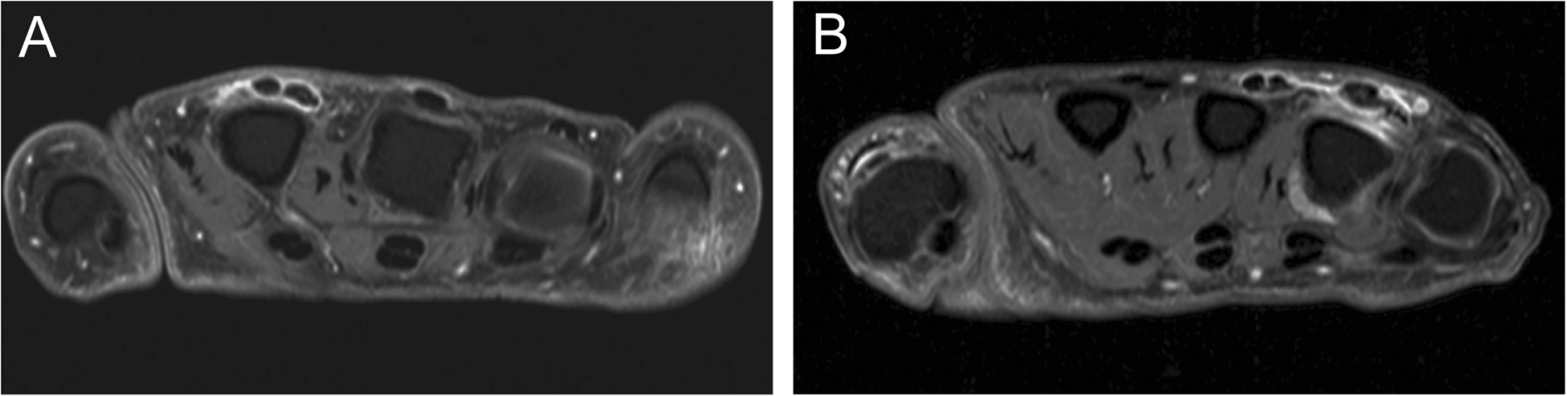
MRI examples of MCP-extensor peritendinitis. MCP-extensor peritendinitis in two CSA patients, depicted in T1-weighted FSE sequences with frequency selective fat saturation in the axial plane of the MCP joints after injection of gadolinium contrast. Patient A had extensor peritendinitis at the level of MCP 2. Patient B had extensor peritendinitis at MCP 4; this patient also had peritendinitis at the level of MCP 3 and synovitis at MCP 4 that was better visualized at adjacent slices
